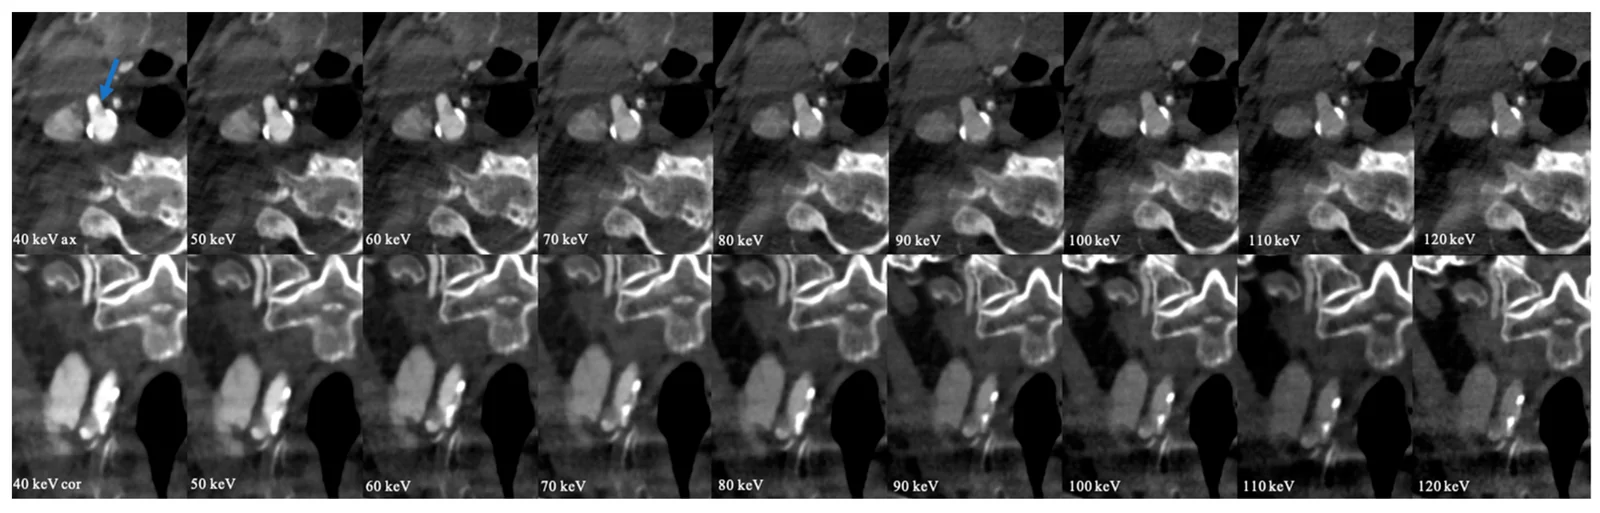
Photon-counting CT (PCCT) virtual monoenergetic images (VMI) of a patient with right-sided carotid atherosclerotic disease. Axial (top row) and coronal (bottom row) reconstructions from 40 to 120 keV. At low keV (40–50 keV), the soft plaque at the carotid bifurcation (blue arrow) is more conspicuous due to increased vascular contrast, but blooming and beam-hardening artifacts from the adjacent calcified plaque are more pronounced, limiting stenosis assessment. At higher keV (70–80 keV), these artifacts are reduced, improving delineation of calcified plaque borders and the vascular lumen.
